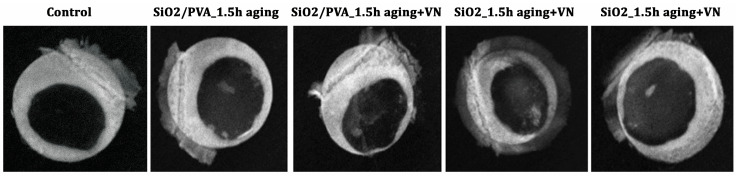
In vivo radiographs of the bone samples after 4 weeks of implantation of silica-based scaffolds.
